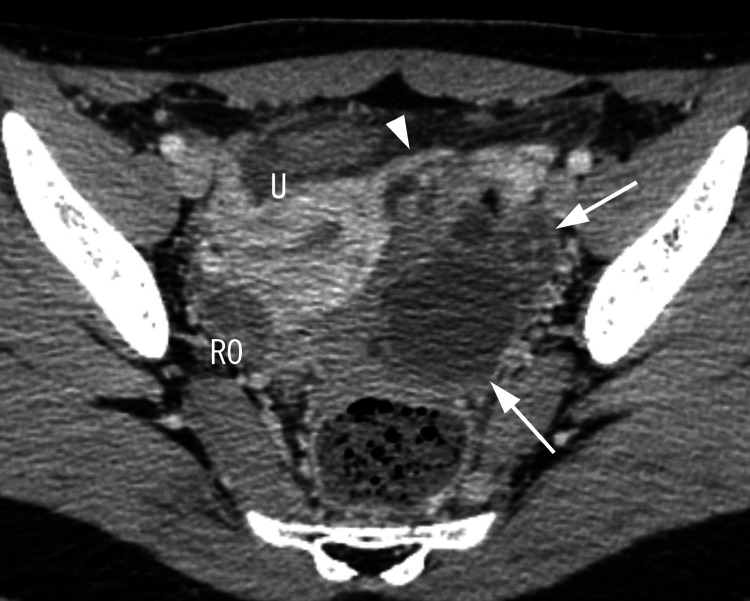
An axial pelvic CT image showing multiple cystic lesions (arrows) in the left adnexa consistent with ovarian torsion. The isthmus of the left fallopian tube was also observed (arrowhead) U: uterus; RO: right ovary.
